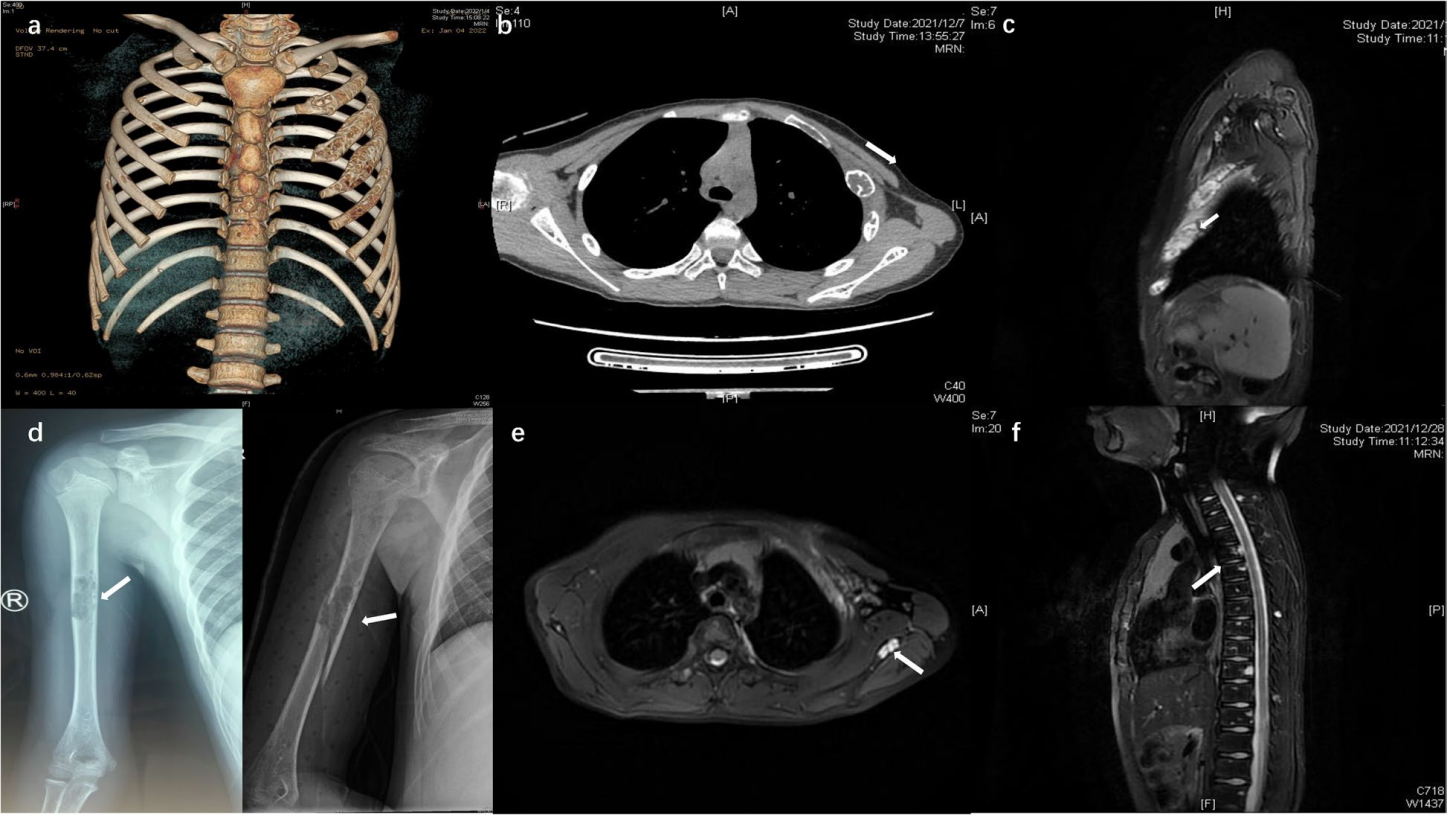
The multiple lesion bone in the left ribs in CT (a, b) and MRI (c). The lesion in the right humerus and pathological fracture (d). MRI scan (T2) showed multiple lytic areas in the scapula (e) and vertebral bodies (f)
